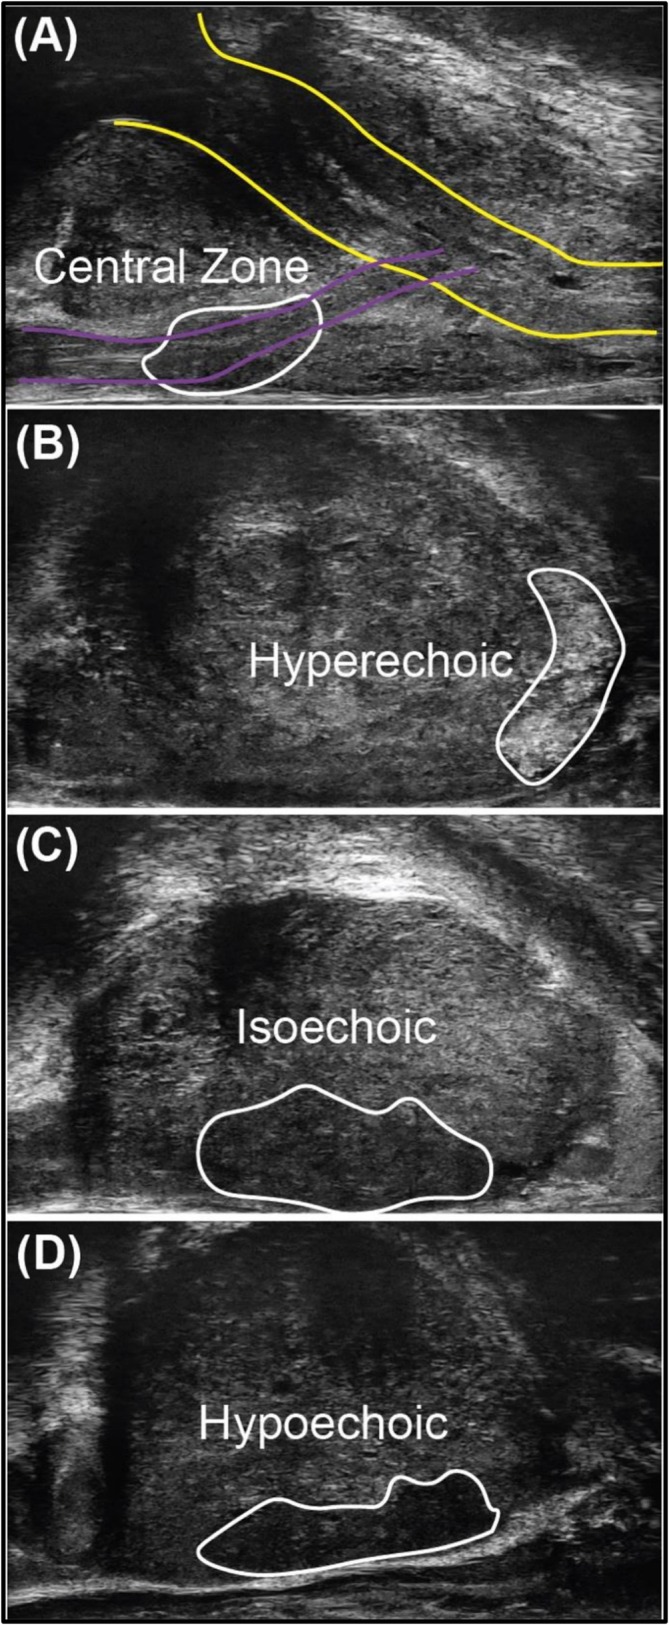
Prostate cancer lesions on micro‐ultrasound exhibiting varying echogenic properties. By default, micro‐US is visualized in the sagittal plane, with the bladder (proximal) on the left of the image, penis (distal) on the right of the image, pubis (anterior) at the top of the image and rectum (posterior) at the bottom of the image. All lesions were biopsied with three target cores. (A) Micro‐ultrasound image with the urethra (yellow), ejaculatory ducts (purple) and central zone (white) displayed in the sagittal plane. (B–D) Micro‐ultrasound lesions with (B) hypoechoic, (C) isoechoic and (D) hypoechoic lesions (white outline).
